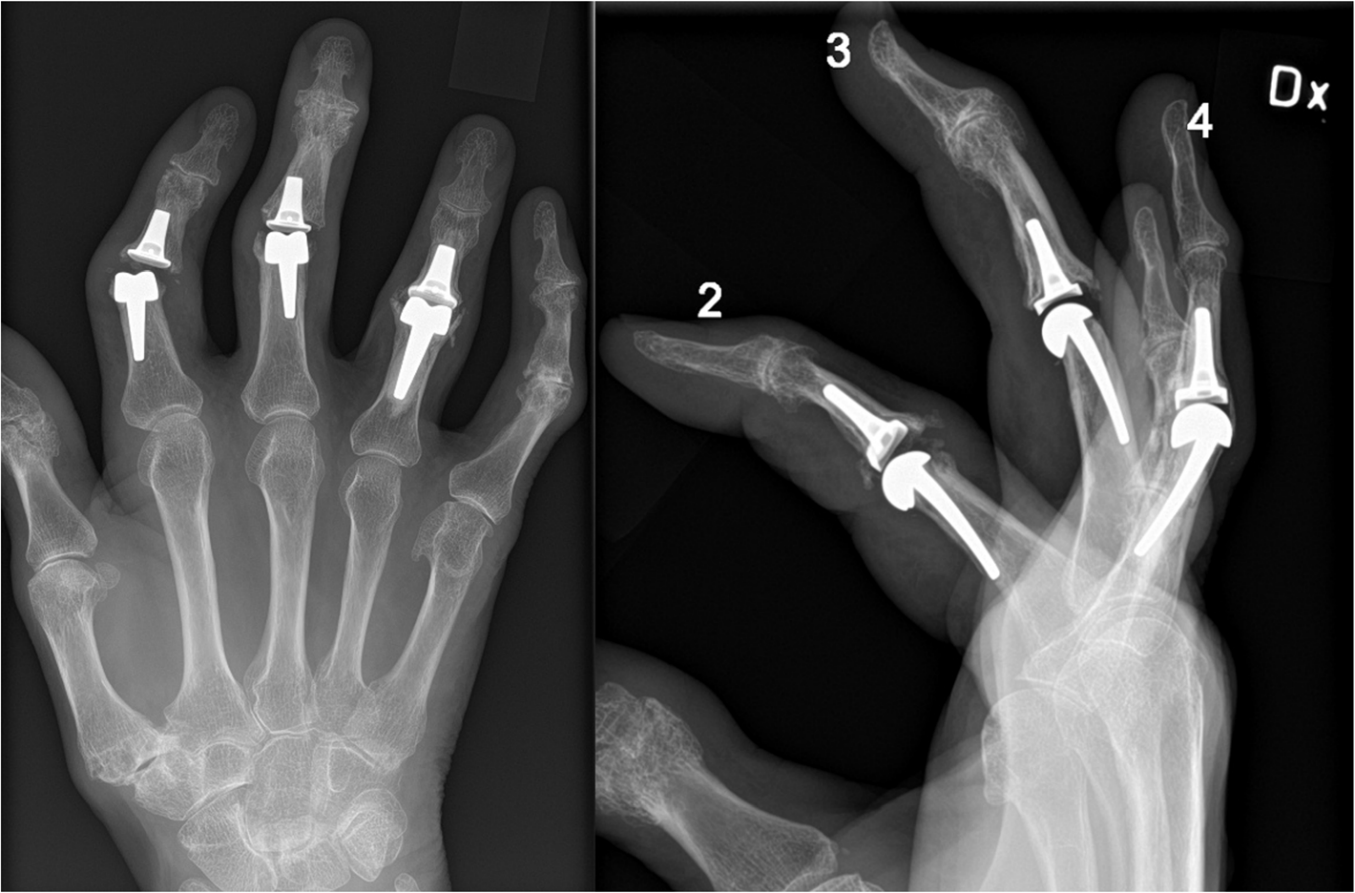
SBI implants at PIP level with different problems. The ring-finger was operated in 2012, and revised in 2015 as the distal component was changed and cemented. Subsequently the joint ended up ankylotic with bony overgrowth. The index and middle-finger were operated in 2016. This radiography from 2017 show signs of subsidence and deviation, especially in the index-finger which later had to be revised and cemented (both components). Only the middle-finger implant has survived without additional surgery
